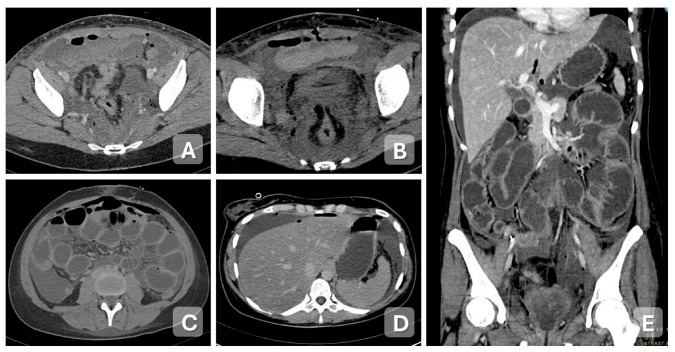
Pelvic inflammatory disease complicated with peritonitis: axial CT with contrast enhancement (A,C,D) and without (B) and coronal (D,E) CT with contrast enhancement showing fluid collections, enhanced peritoneal lining, fat stranding, inflamed bowel loops, pneumoperitoneum, and fatty infiltration around the bowel.
